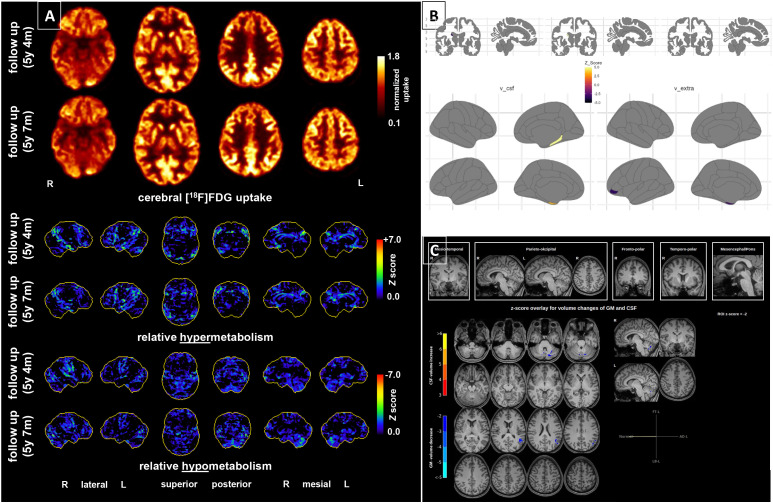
Brain imaging findings during the relapse five and a half years after the first psychotic episode. (A) [^18^F]Fluorodeoxyglucose (FDG) positron emission tomography (PET) in the second episode (before steroids) revealed low to moderate hypermetabolism of the association cortices and cerebellum. The follow-up did not show relevant improvement in the findings. R=right, L=left. (B) Advanced magnetic resonance imaging (MRI) with diffusion microstructure imaging (DMI) in the second episode (before steroids) showed frontal and thalamic microstructural alterations of grey matter integrity (comparison of the studied patient with 24 sex- and age-matched healthy controls, mean age 27.38 ± 4.48 years. Data were parcellated according to the ASEG atlas (top row) and the Desikan-Killiany (bottom row). The cerebral blood flow using arterial spin labeling MRI was normal (not shown). v=volume. (C) MRI showed no macrostructural volume loss (https://www.veobrain.com/?page=veomorph) in the second episode.
